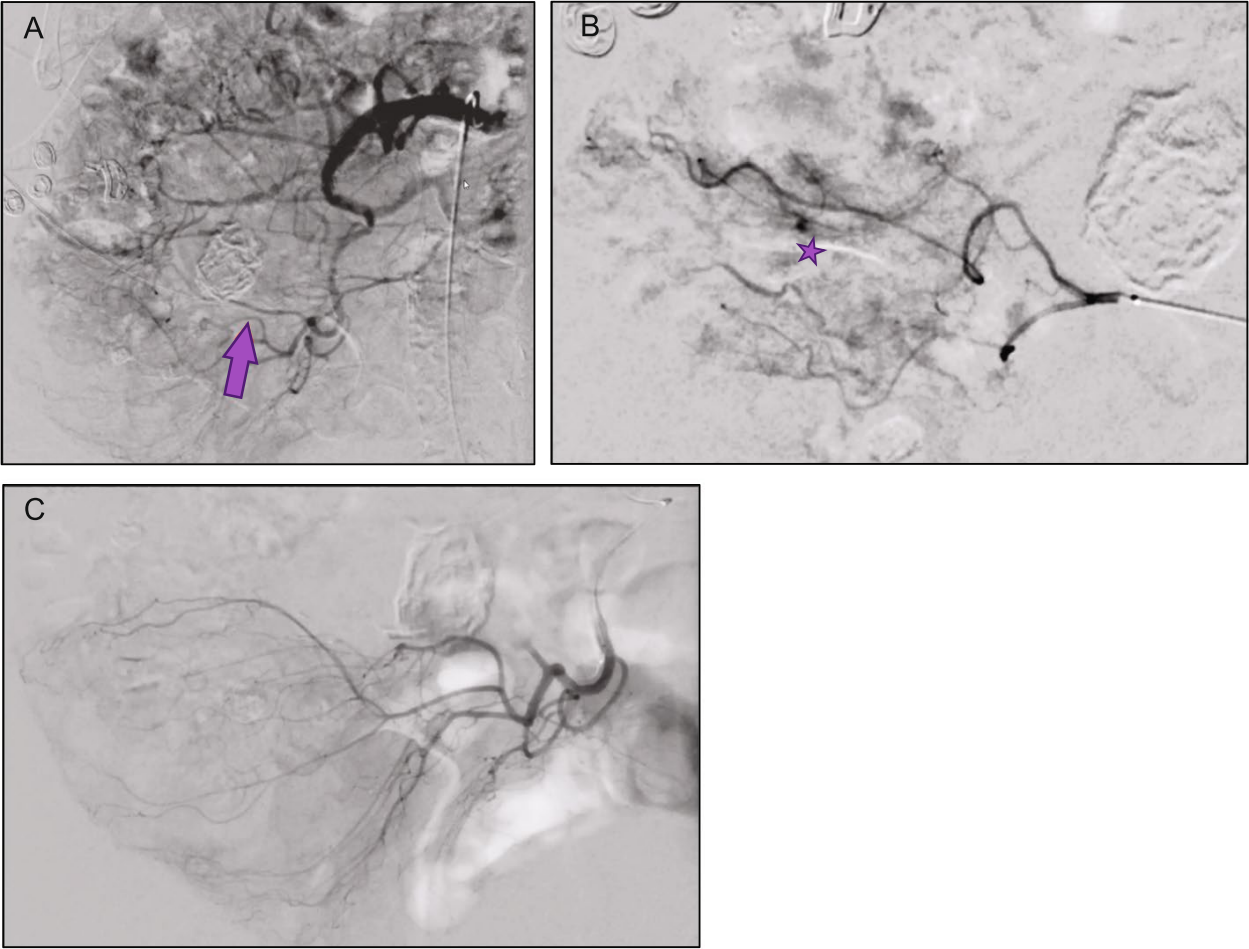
Case example 3: Lower GI bleed—pseudoaneurysm. The patient presented with hypotension and hematochezia. Emergency CT and angiograms identified a tortuous superior mesenteric artery (A) and small terminal branch pseudoaneurysm (PSA) (B). The terminal cecal branch was embolized using 0.2 cc of Obsidio, with slow injection force to allow embolic distal and proximal to the pseudoaneurysm. The PSA was completely embolized while still providing robust blood flow to the cecum (C)
